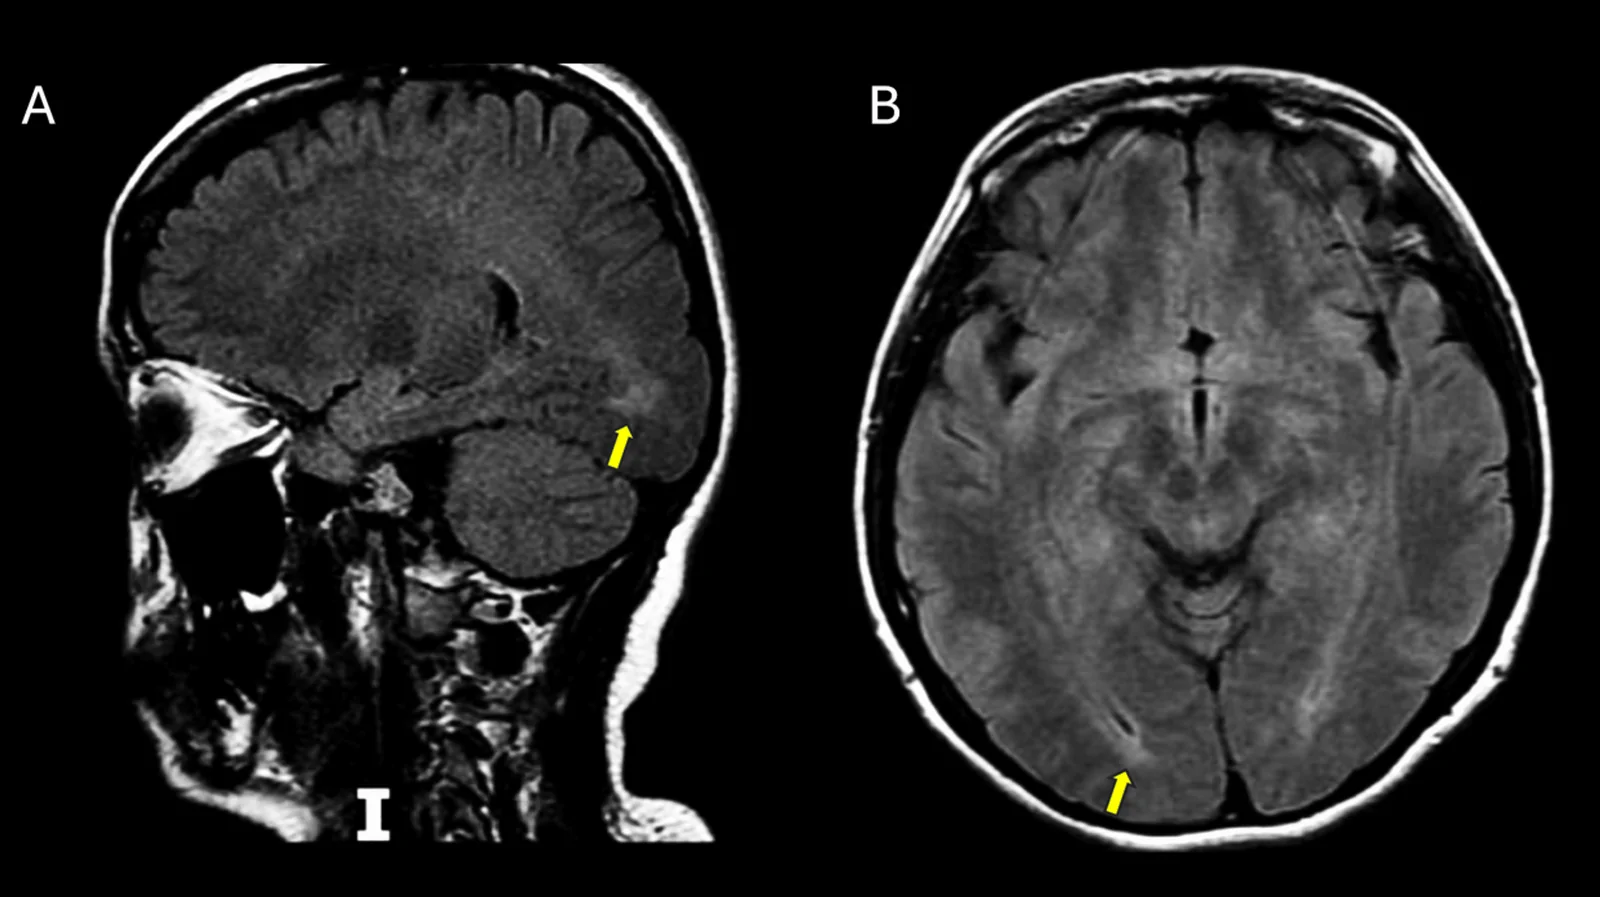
Brain MRI findings. (A) Sagittal FLAIR and (B) axial FLAIR images showing nonspecific occipital/subcortical white matter hyperintensities. Yellow arrows indicate areas of abnormal signal. FLAIR: Fluid-attenuated inversion recovery.
